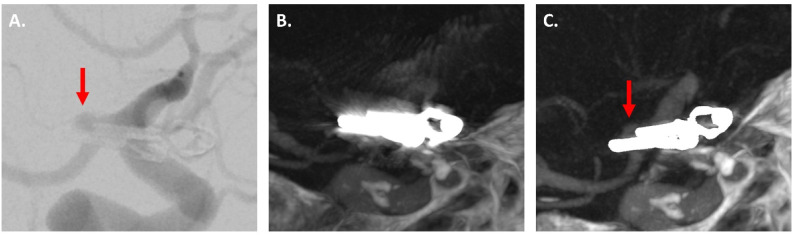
An 80-year-old patient with an aneurysm of the right posterior communicating artery treated via intracranial clipping. DSA control revealed a small residuum at the aneurysm neck ((A) red arrow). The aneurysm was not assessable in iMAR− FD-CTA due to severe metal artifacts (B). After application of iMAR and the following significant metal artifact reduction, FD-CTA also demonstrated the small residuum ((C) red arrow).
